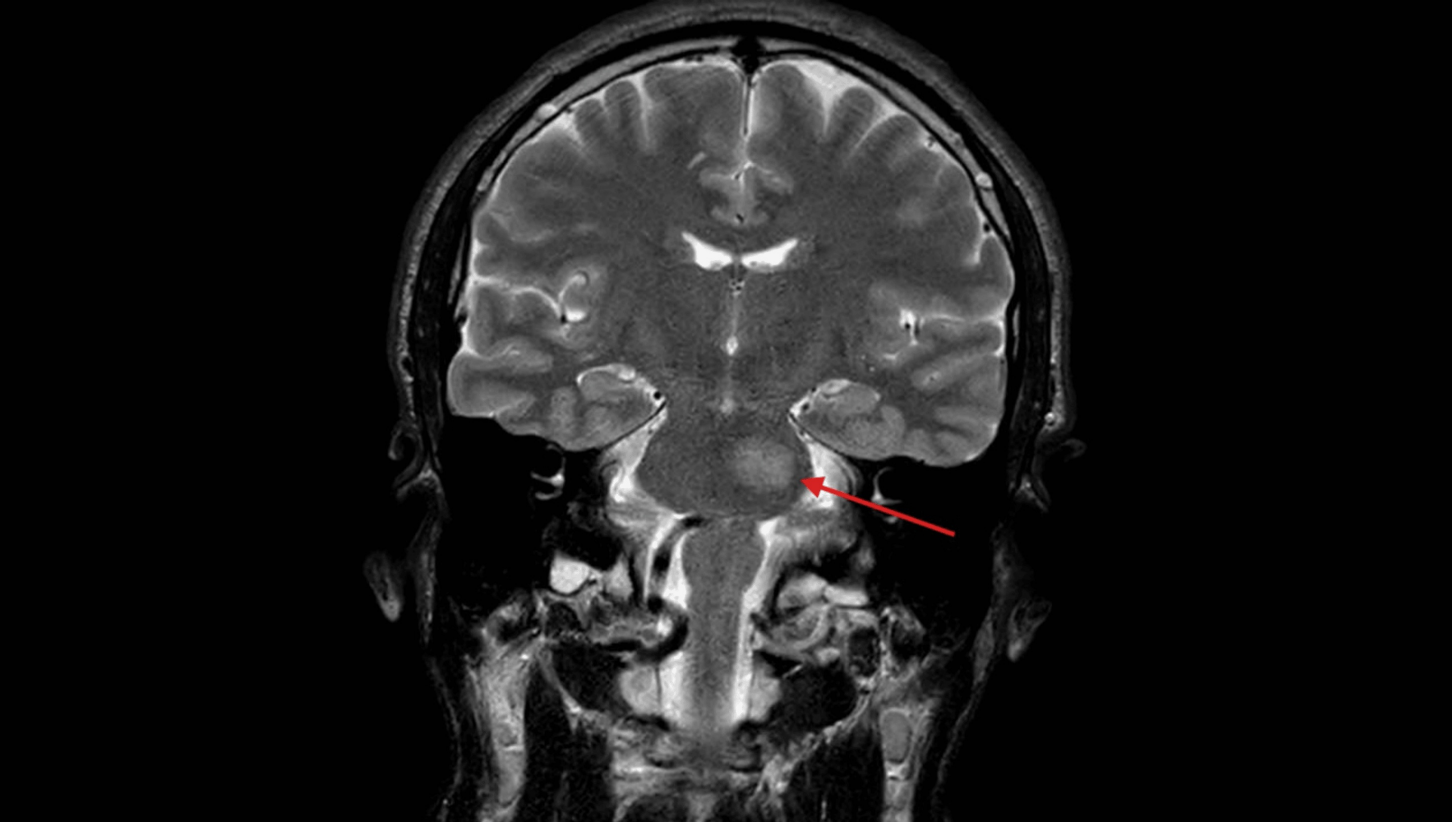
Coronal T2-weighted MRI showing a T2-hyperintense, expansile demyelinating lesion in the right pons, as indicated by the red arrow. The lesion extends toward the right middle cerebellar peduncle.
